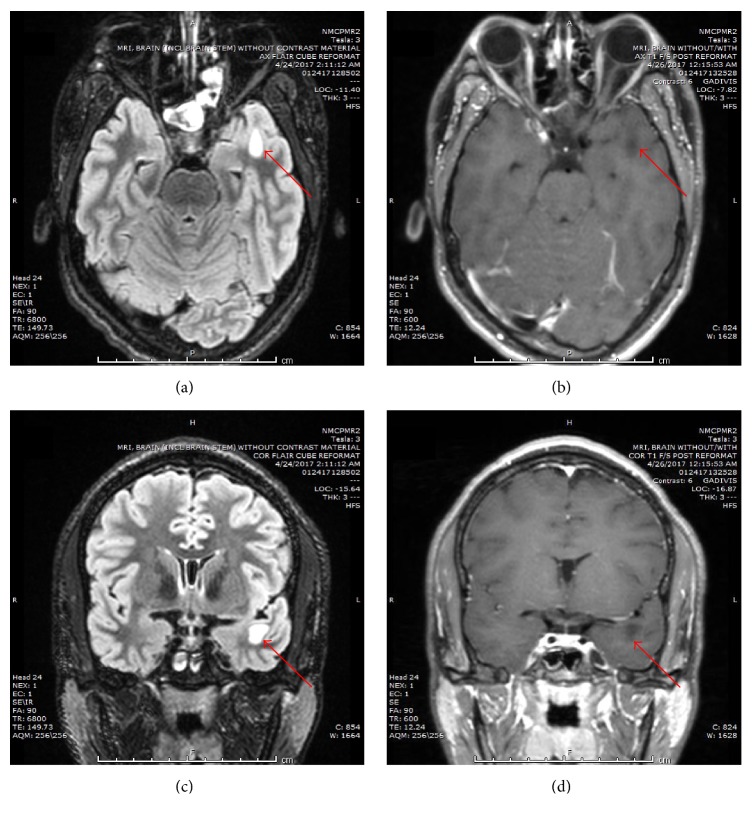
Brain MRI demonstrating left temporal lobe lesion. (a, c) Axial and coronal T2 weighted images. (b, d) Axial and coronal T1 weighted images. Red arrow points to lesion.
